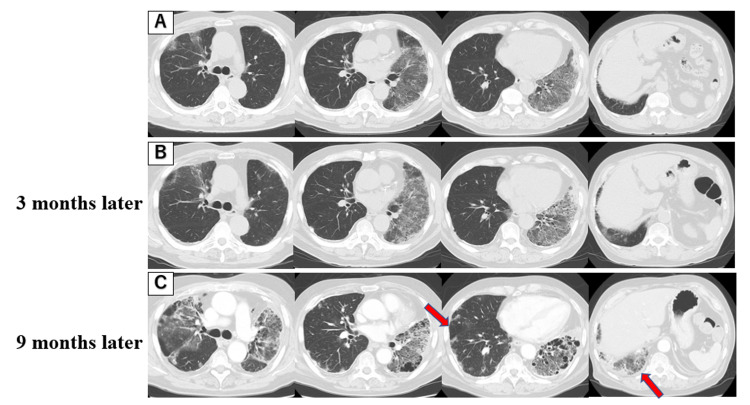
Chest CT images of the patient over time and location of partial lung resection. The resection site was described by the bronchopulmonary segments (S1-10). (A–C) The chest CT showed multiple GGOs that spread over time. Partial lung resection was performed from two locations (arrows): from the right middle lobe (S4), which was near normal grossly, and the right lower lobe (S10), which was highly fibrotic.
